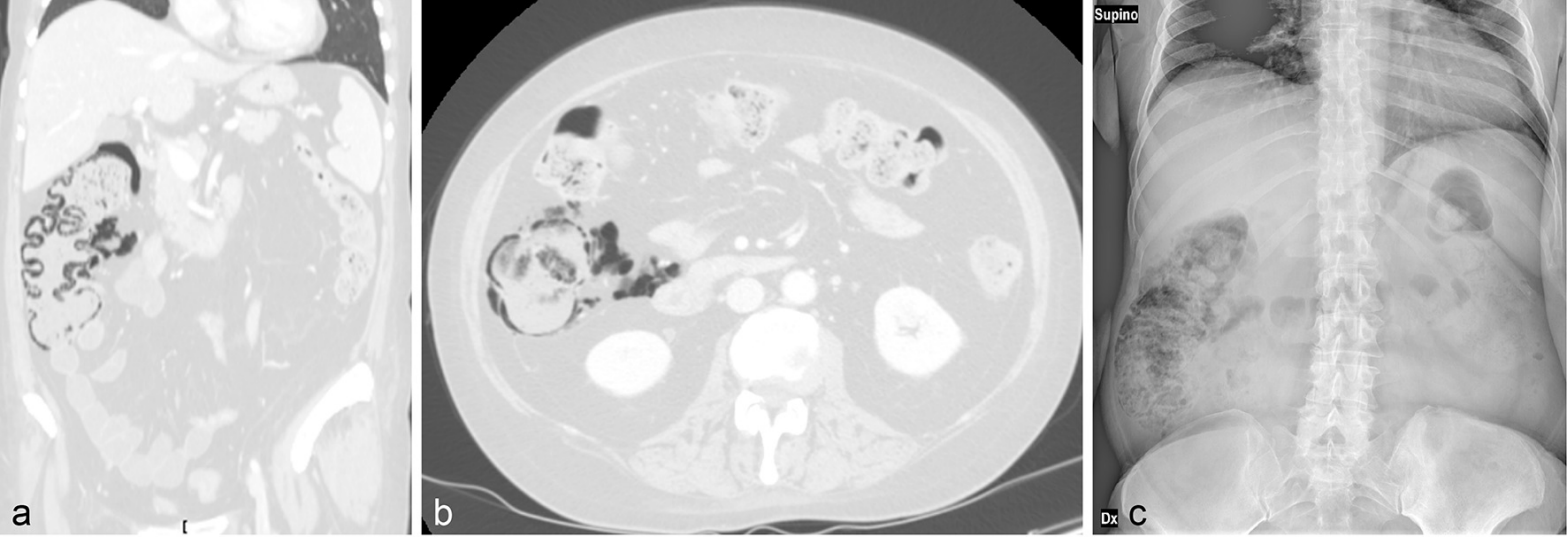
Case 1. A 56-year-old female with metastatic (liver, bones and brain) breast cancer receiving systemic chemotherapy plus corticosteroids. Restaging body CT scan, coronal (a) and axial (b) reformatted images; lung window. There is a moderate amount of pneumatosis in the mesentery and pericolic fat , and intramural gas along the caecum, ascending colon and a short section of the transverse colon. Bowel walls are not thickened or distended, haustra are regular and mesenteric vessels are patent. A plain abdominal film (c) taken 5 days later shows persistent asymptomatic pneumatosis.
